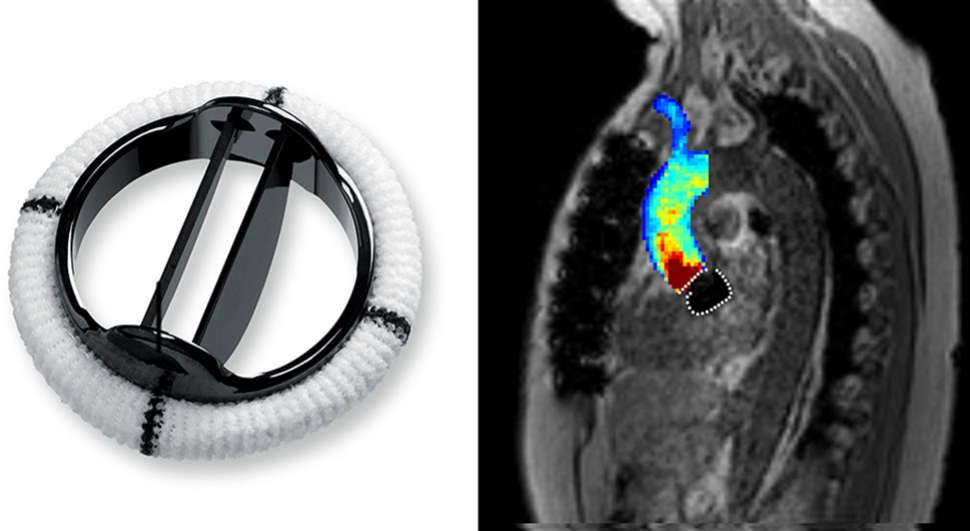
Examples of a mechanical aortic valve prosthesis (left, with permission of Abbott) and a velocity maximum intensity projection measured with 4D flow MRI (right). Marked white delineated area (right) represents susceptibility artifact caused by mechanical valve prosthesis
